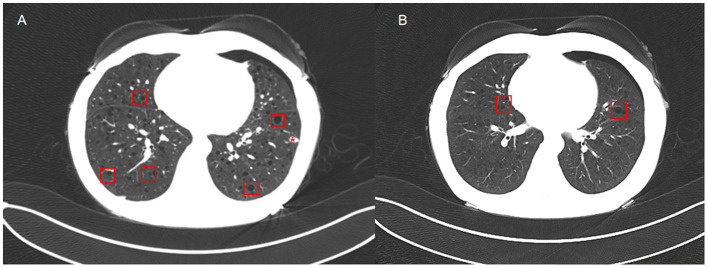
(A, B) High-resolution computed tomography (HRCT) shows multiple diffuse round small cystic spaces in both lungs at different levels, with uniform distribution and varying sizes. “□” indicates dilated small cystic spaces, and “*” indicates pulmonary tuberculosis nodules.
